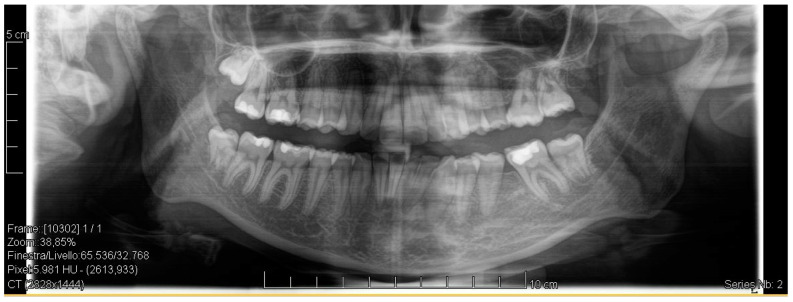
Post-treatment radiograph showing extractive spaces’ closure.
